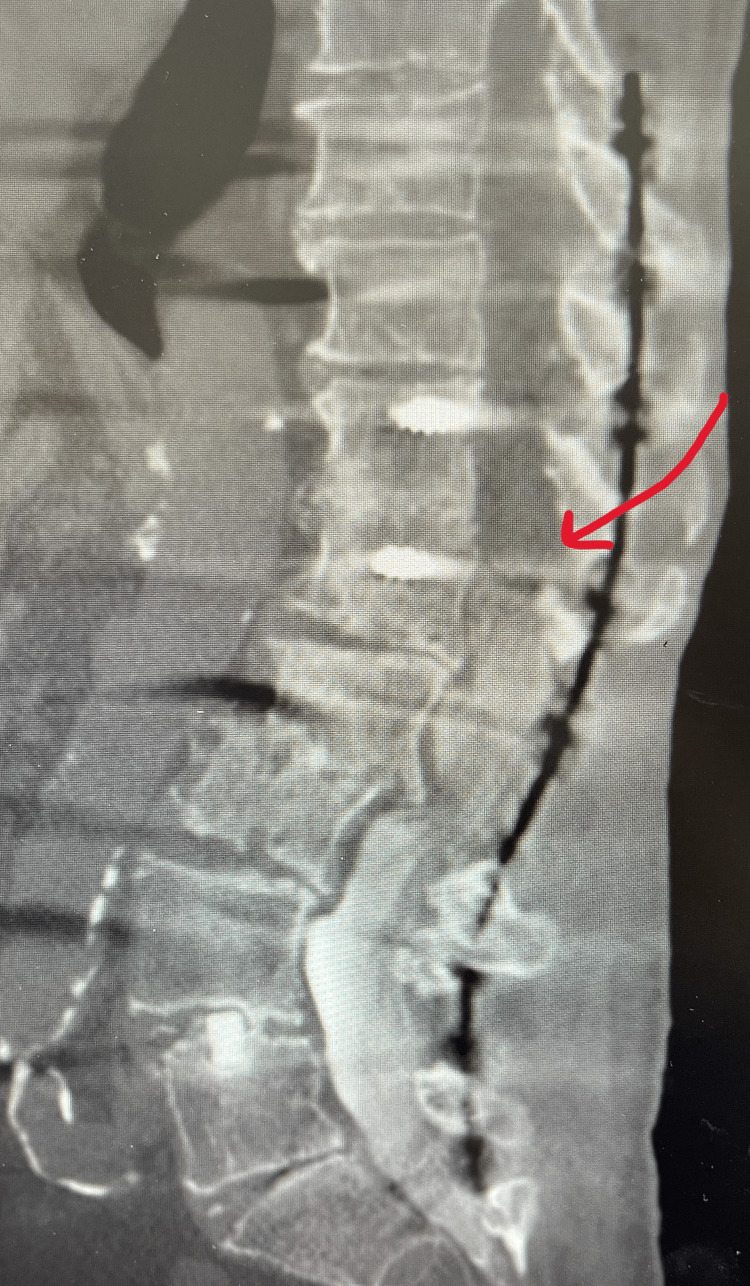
Limited lateral CT myelogram imaging study with IV contrast blockage at L1 (red arrow)
